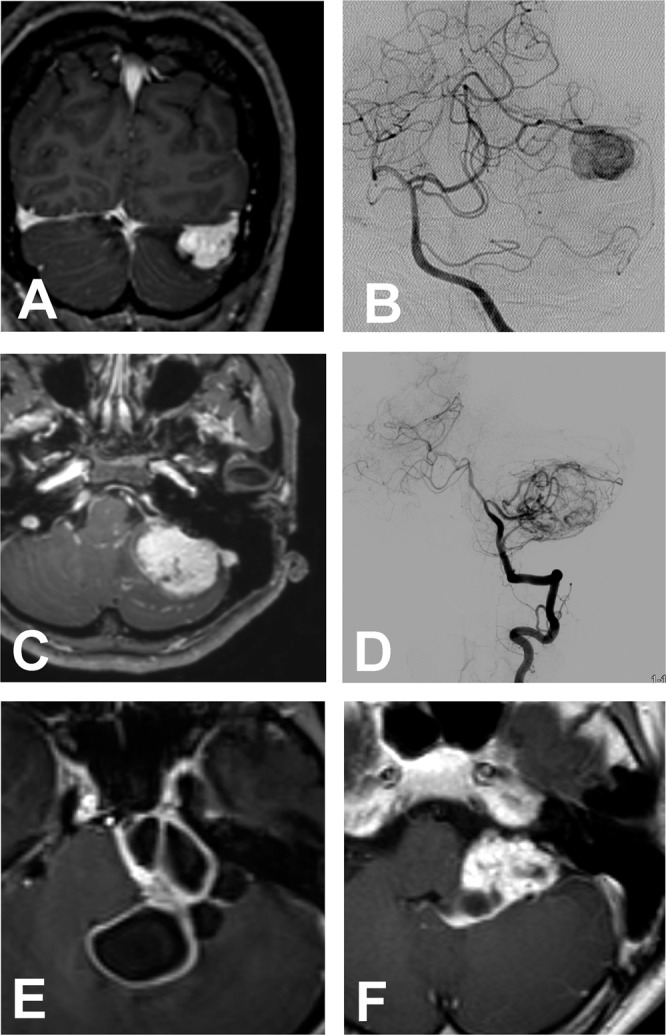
Hemangioblastomas (HBMs) showing unusual radiological findings on Gadolinium-enhanced T1W images of magnetic resonance imaging. Solid hemangioblastomas (Type S) are located on the hemispheric cortex (A) and petrous surface (C) that mimic meningiomas. Cerebral angiography revealed that these tumors were fed by superior cerebellar artery (B) and anterior inferior cerebellar artery (D), respectively, which was very helpful in differential diagnosis. A multiple intra-tumoral cystic HBM (Type Ci) is exophyting into a cerebellopontine and cerebellomedullary cistern (E and F).
